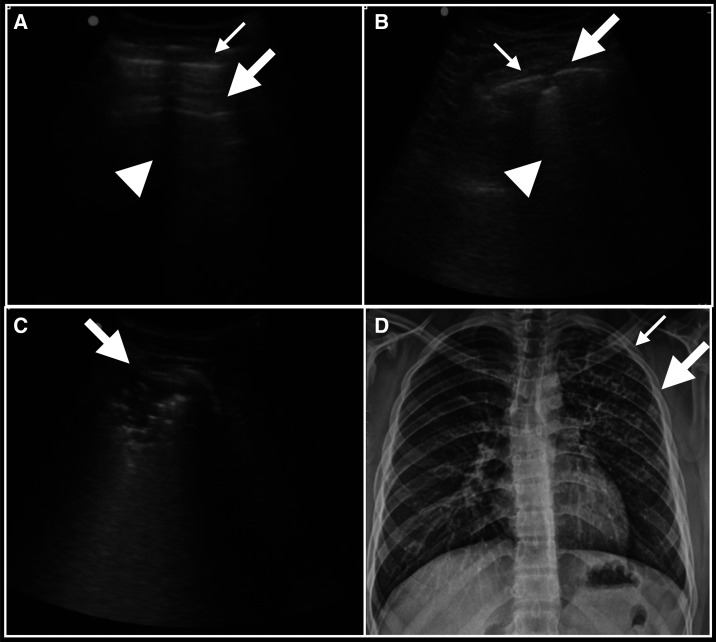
Typical appearance of lesions in lung ultrasound (LUS) and chest X-ray from a pulmonary tuberculosis (PTB) patient: (A) *Normal* LUS with white pleural line (thin arrow) and A-line reverberation artifact (thick arrow), indicative of normal air-filled pulmonary parenchyma. Rib shadow (arrow head) in the center. (B) Typical appearance of small subpleural consolidation (SPC) (thick arrow),characterized by subpleural hypoechoic region < 1 × 1 cm, with distinct borders and trailing artifact (arrowhead), next to the normal white pleural line (thin arrow). (C) Typical appearance of consolidation (thick arrow), characterized by echo-poor region > 1 × 1 cm, with or without air bronchograms. Air bronchograms seen here as hyperechoic spots within the consolidation. (D) Chest X-ray from a PTB patient demonstrates patchy fibronodular left upper lung field consolidation (thick arrow) and cavity (thin arrow). Lung ultrasound of the same patient showed multiple SPCs throughout the left upper and middle hemithorax, and a 3.5 × 1.2-cm consolidation in the left middle lung field.
